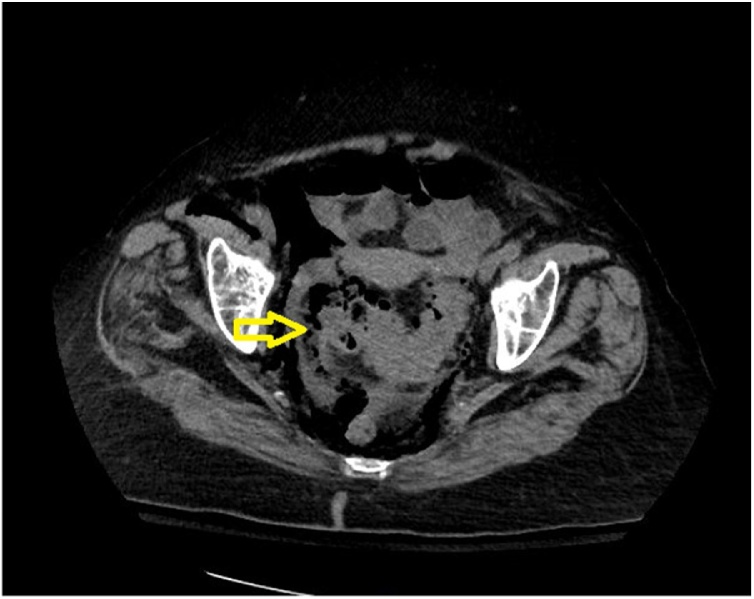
Thickened rectosigmoid with surrounding air. Coronal plane CT scans abdomen immediately following colonoscopy indicating thickened rectosigmoid with surrounding air (Yellow arrow).
